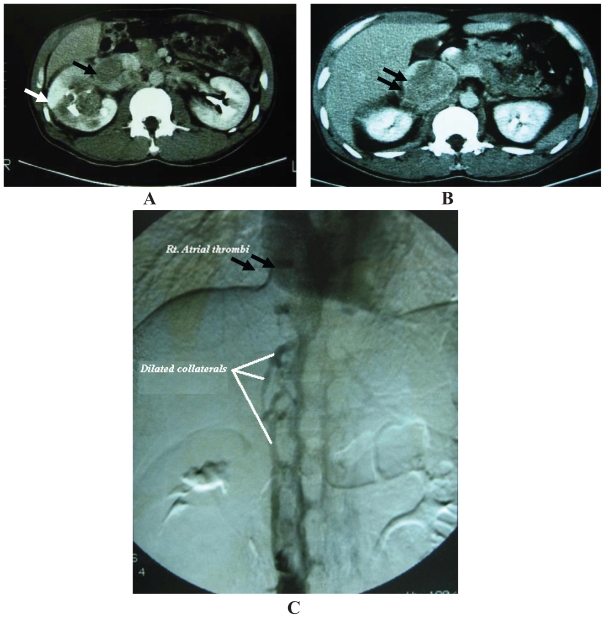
A: Abdominal CT showing Rt. RCC (white arrow) with a large tumor thrombus extending into the IVC (black arrow). B: Abdominal CT for the same patient’s Rt. kidney (Upper pole) with a large tumor thrombus extending more cephalad in the IVC (black arrow). C: Venacavography (for the same patient) showing dilated IVC collaterals due to a large tumor thrombus extending into the IVC (white arrows) up to right atrium (black arrows).
